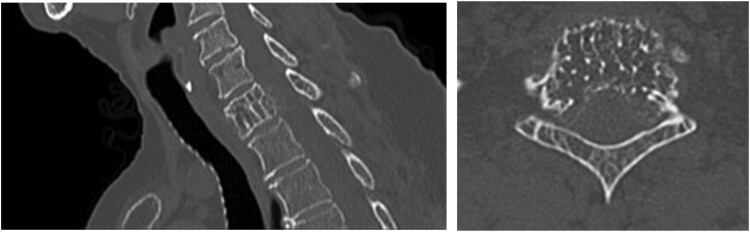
CT scan in sagittal and axial views through the first thoracic vertebra. The components which extend into the epidural space, bilateral T1 neural exit foramen and inferomedial aspect of the left C8 neural foramen contain thin calcific/ossific trabeculations. Vertebral height is maintained, and alignment is normal.
